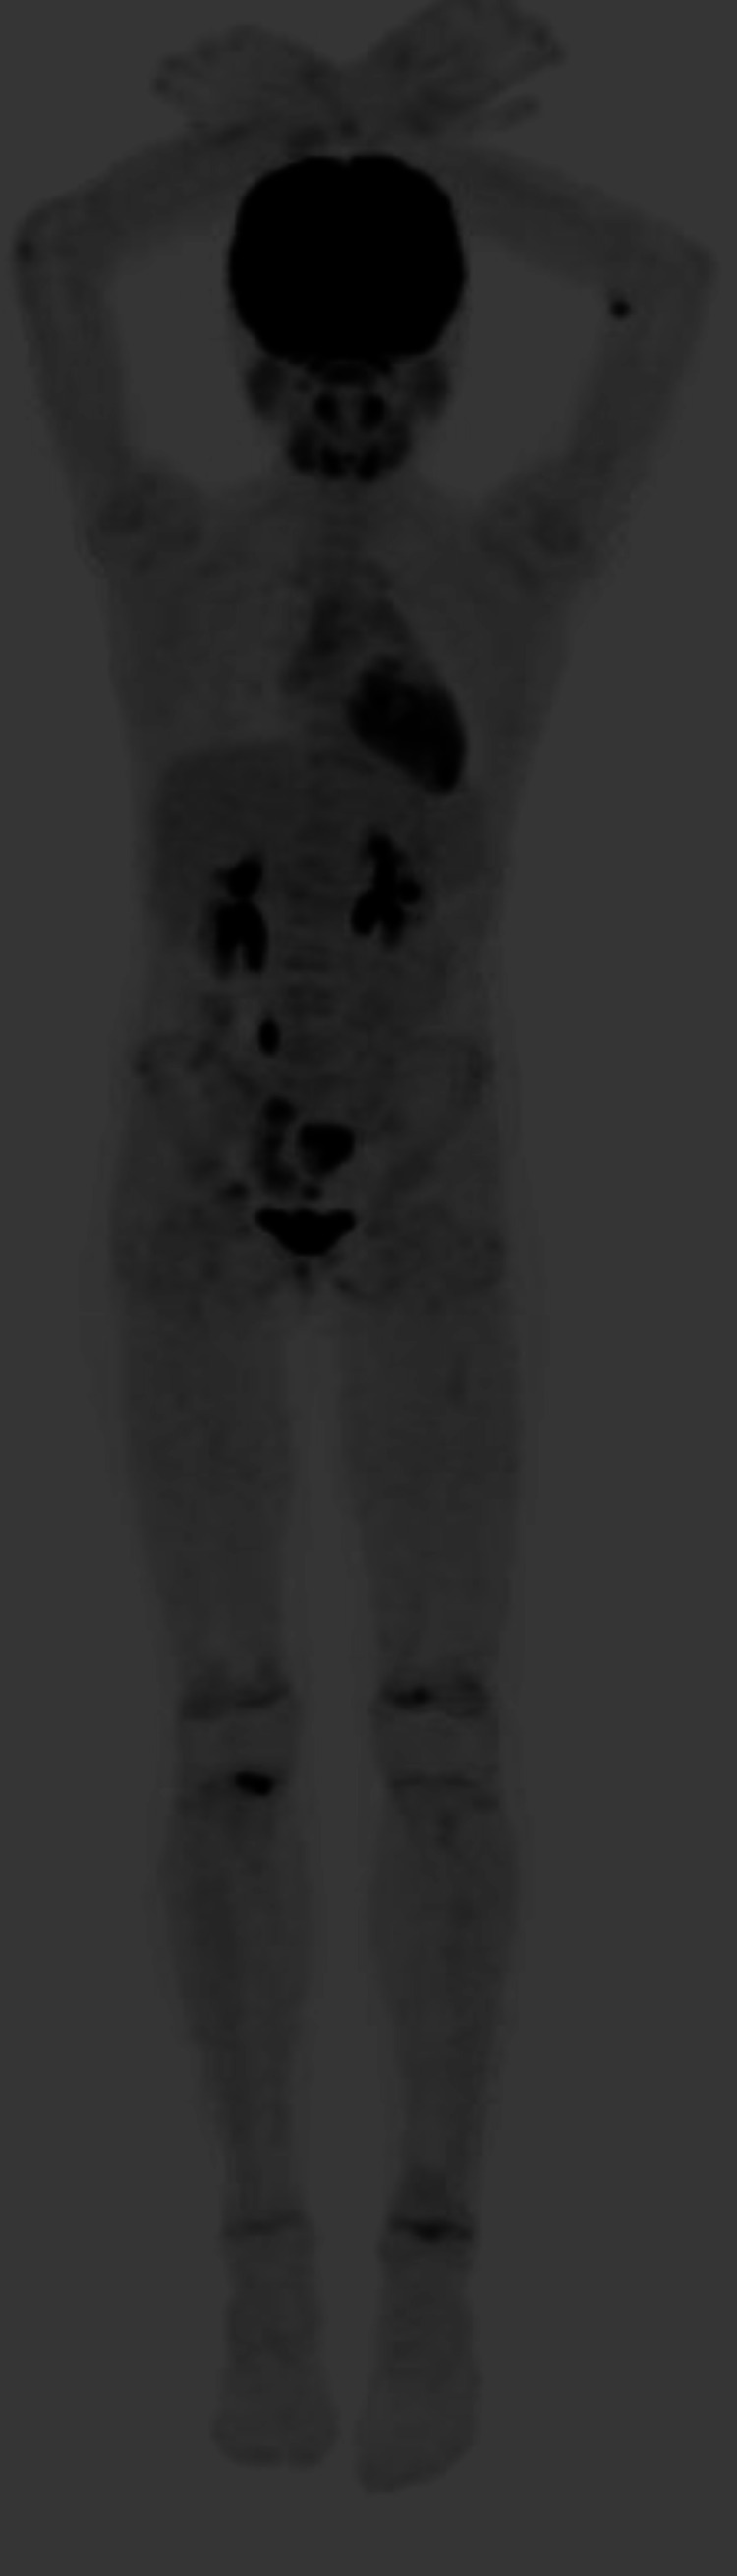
PET CT scan using F-18 fludeoxyglucose to highlight areas of increased metabolic activity. Metabolically active lytic osseous lesions can be found within the axial and appendicular skeleton.
